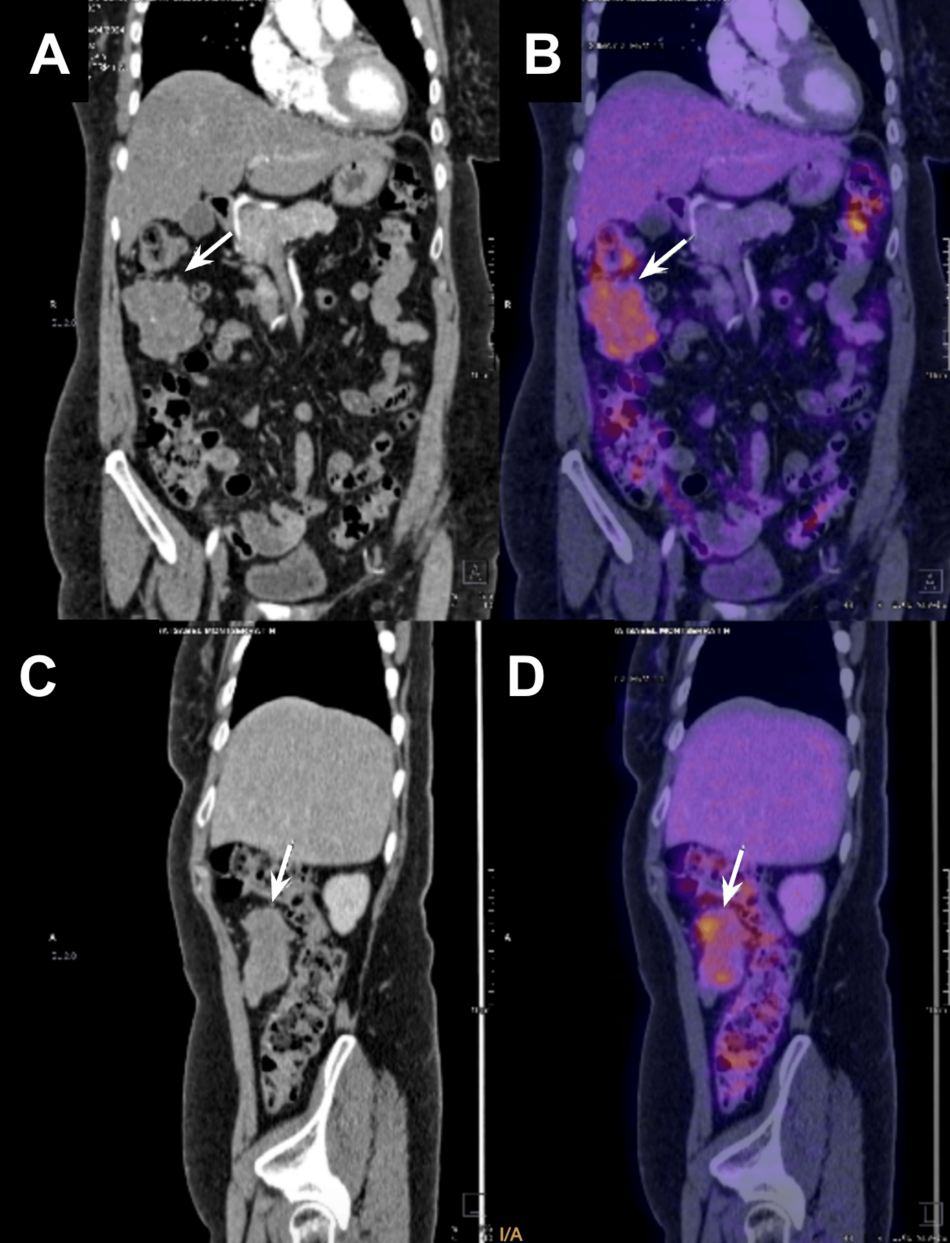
Preoperative PET-CT scan coronal and sagittal view. Preoperative PET-CT scan presenting a solid peritoneal mass measuring 5.8 x 3.5 cm (white arrow) in the anterior right flank. A) Coronal view of the peritoneal mass (white arrow) adjacent to the ascending colon below the right kidney. B) Coronal view of the peritoneal mass (white arrow) exhibiting an average attenuation of 37 HU. C) Sagittal view of the peritoneal mass (white arrow) adjacent to the ascending colon at the level of the lower pole of the right kidney. D) Sagittal view of the peritoneal mass (white arrow) exhibiting an enhancement up to 60 HU in the venous phase.
